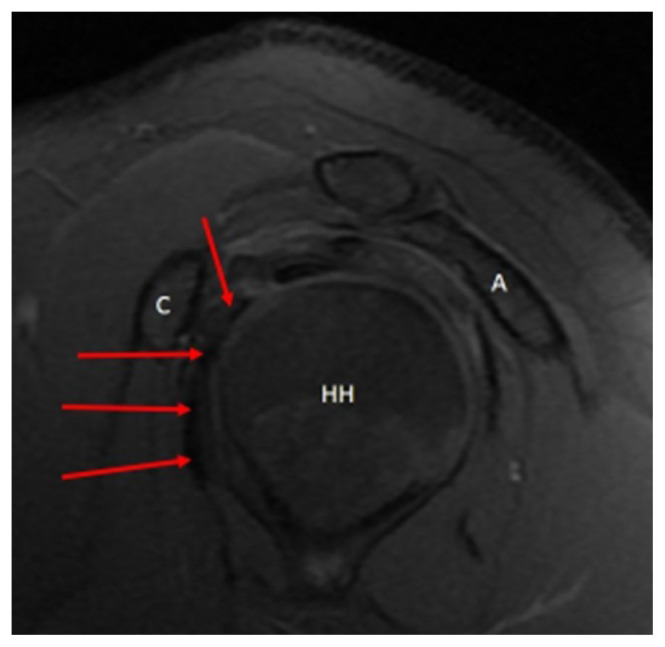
Subscapularis tendon slip number (STSN-red arrows) on sagittal oblique sections of magnetic resonance imaging scans.
